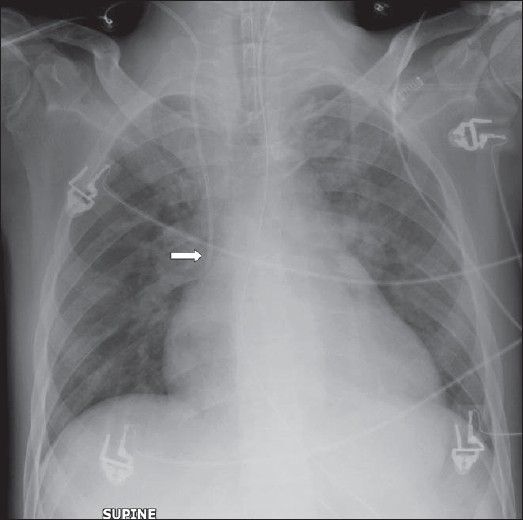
Radiograph showing the tip of an intravenous line to lie at the junction of the superior vena cava and the left atrium
